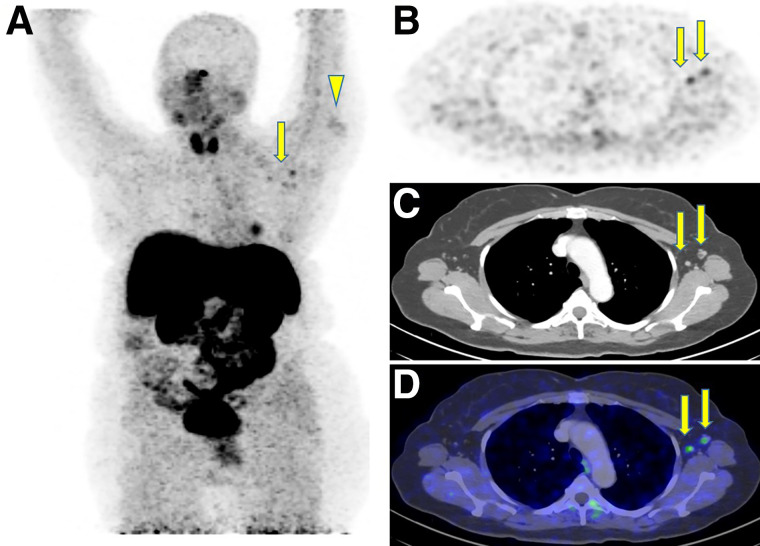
^68^Ga-DOTATATE PET/CT after COVID-19 vaccination in 68-y-old woman with newly diagnosed typical carcinoid (G2), not currently receiving immunosuppressive treatment: maximal-intensity projection (A), axial multiplanar reformation (B), contrast-enhanced CT (C), and PET/CT (D) 24 d after first vaccine and 3 d after second vaccine, both on left side. Increased uptake was observed in left deltoid muscle (arrowhead), corresponding to injection site, and in left axillary lymph nodes of normal size (arrows).
